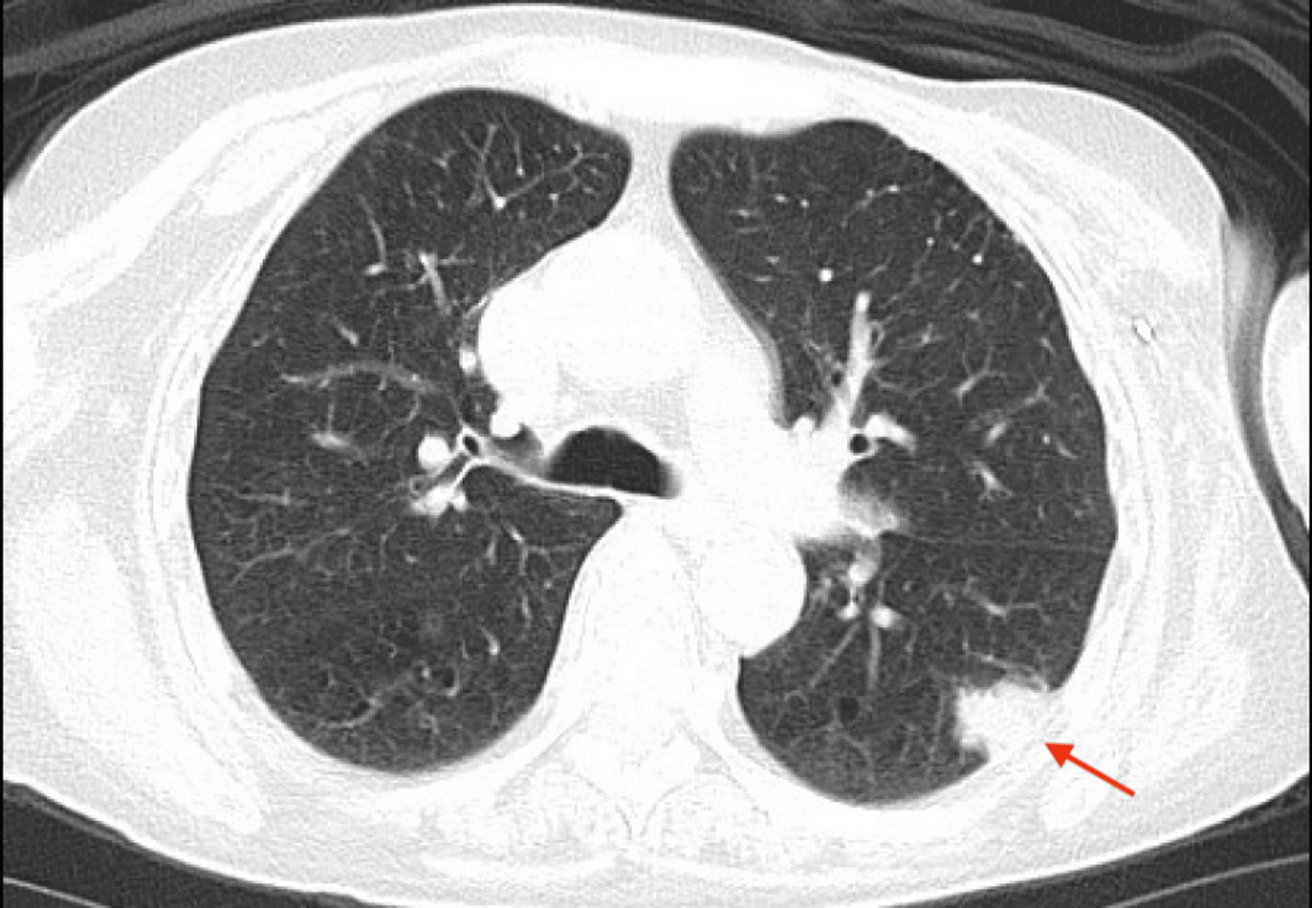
A computed tomography (CT) scan of the chest demonstrating a 1.9 cm left lower lobe pleural-based solid nodule as indicated by the red arrow.
